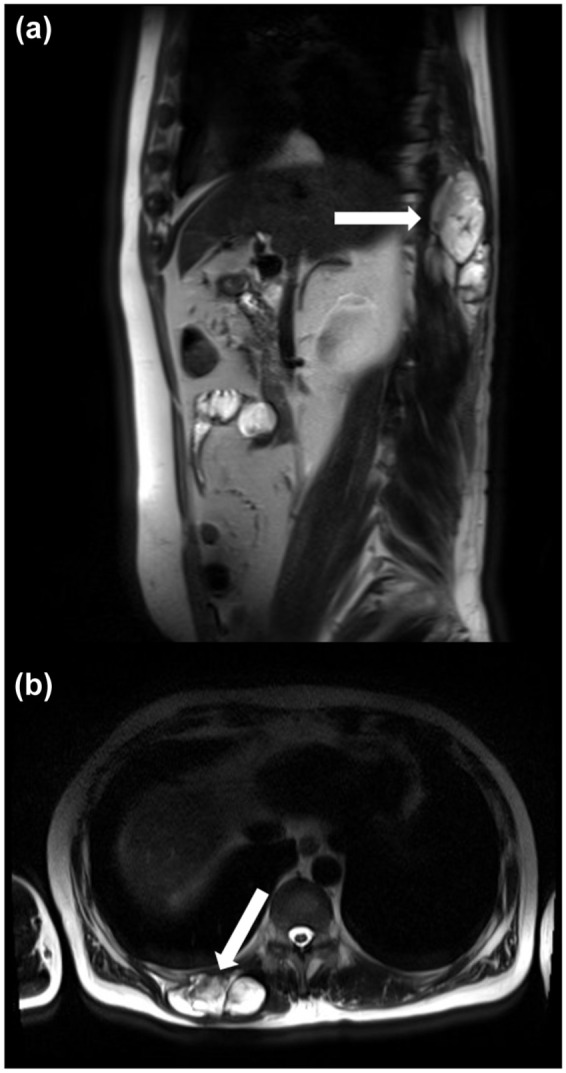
The MRI contrast-enhanced image of myxofibrosarcoma: (a) axial view and (b) sagittal view. MRI-magnetic resonance imaging.
